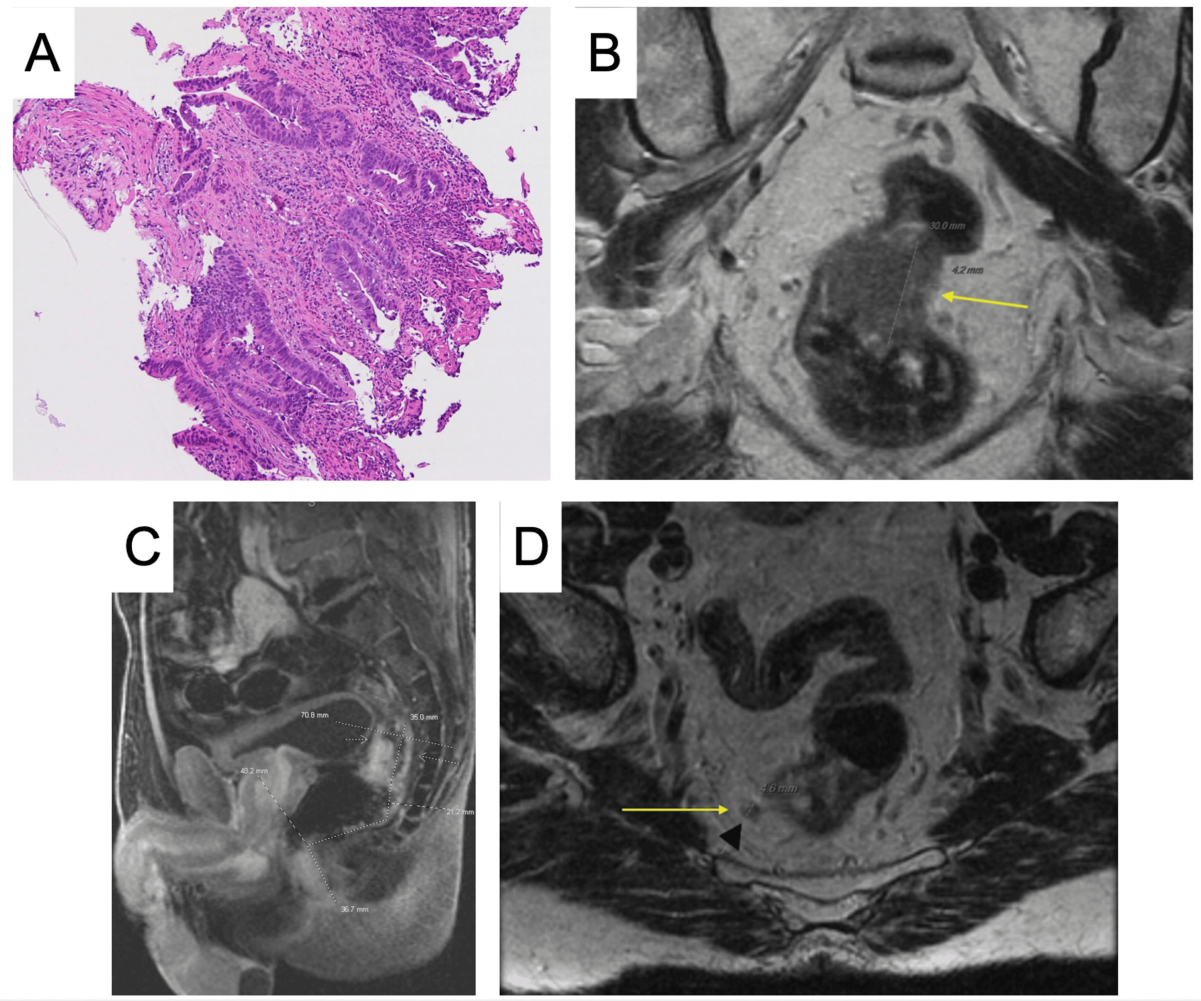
(A) Pathological evaluation of moderately differentiated invasive colonic adenocarcinoma. (B) MRI demonstrating a 3 cm non-mucinous semi-circumferential lesion involving the anterior rectal wall with a 4 mm extramural depth of invasion. (C) Sagittal view of an MRI of the abdomen and pelvis. (D) MRI demonstrating an involved mesorectal lymph node measuring 4.6 mm. MRI: magnetic resonance imaging
